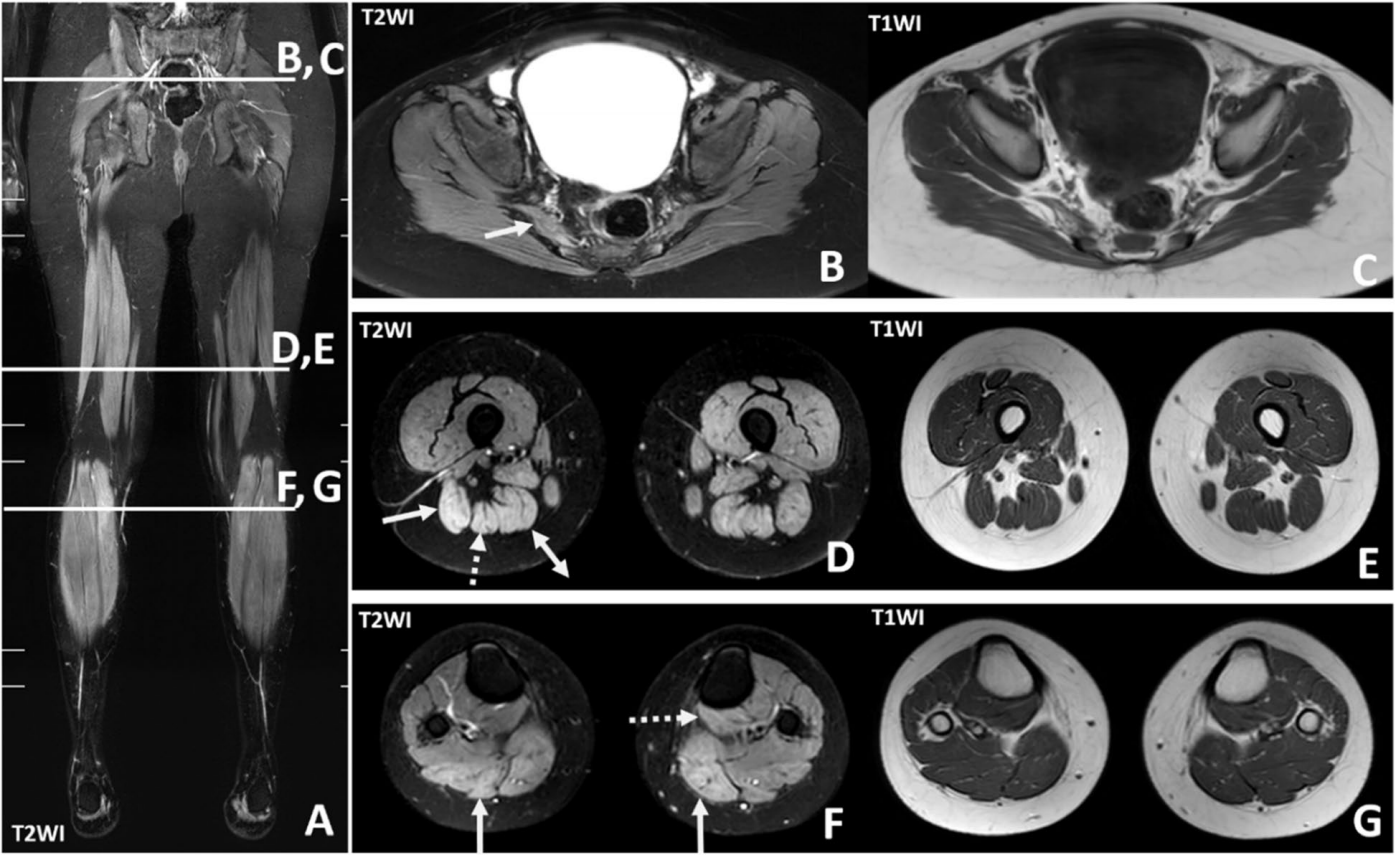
Magnetic resonance imaging (MRI) of lower extremities. A Coronal T2-weighted image (T2WI) B, D, F: Axial T2WI and C, E, G: Axial T1WI. The axial and coronal images show increased signal intensity on T2WI of right piriformis muscle (solid arrow in B), right long head of biceps femoris muscle (solid arrow in D), right semitendinosus muscle (dash arrow in D), right semimembranosus muscle (double-head arrow in D), bilateral gastrocnemius muscles (solid arrow in F) and left popliteus muscle (dash arrow in F)
